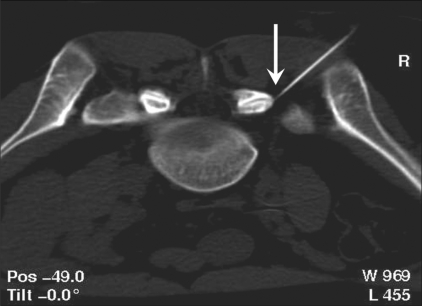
CT-guided facet injection. Axial CT scan in the prone position shows the needle positioned at the lip of the right L5–S1 facet joint.
